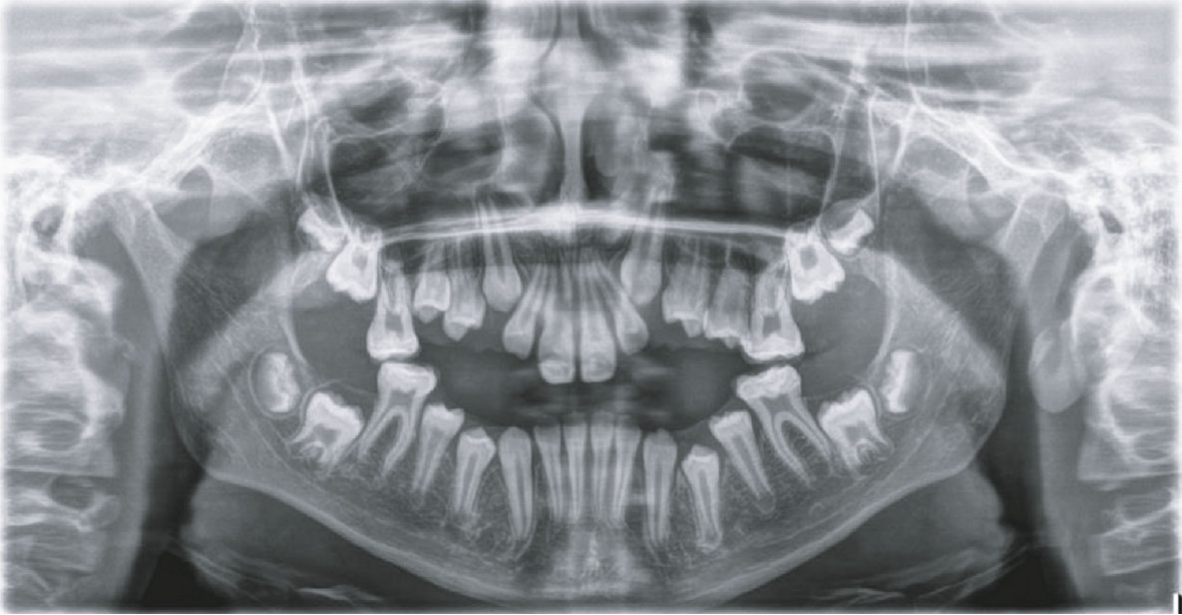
Preoperative panoramic radiographic view.
